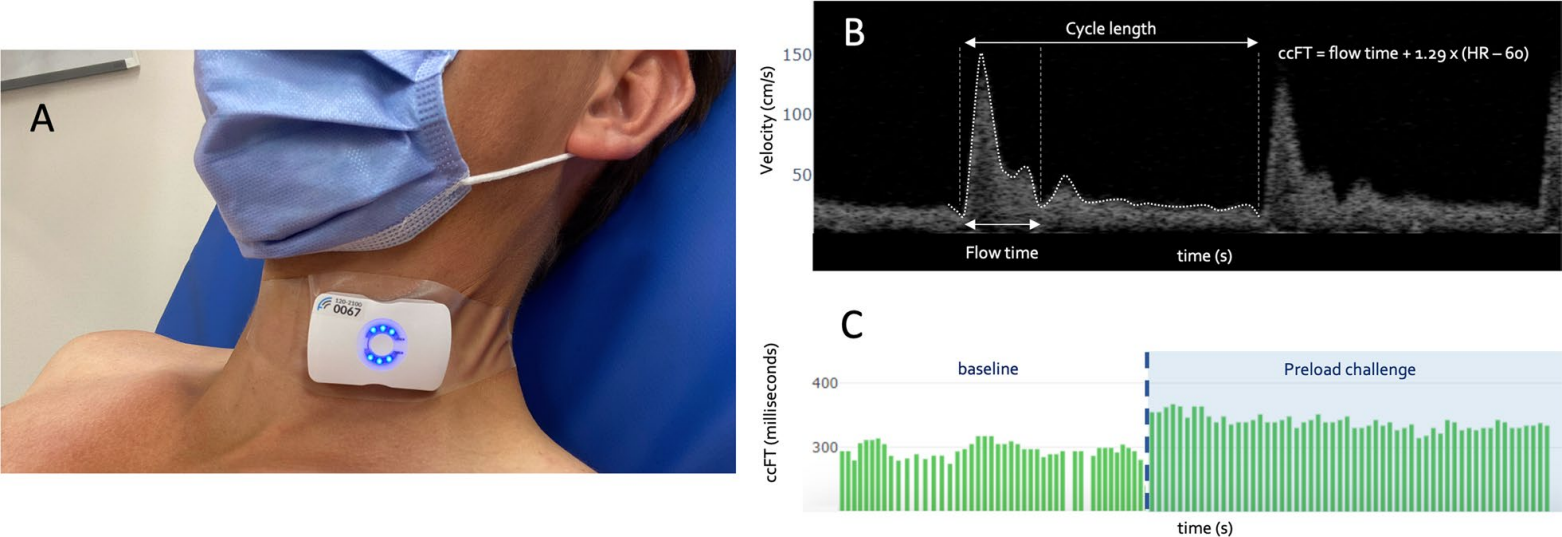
The wearable Doppler ultrasound and its interface. A The device on a healthy volunteer. B Carotid Doppler spectra showing calculation of carotid corrected flow time (ccFT) by the equation of Wodey. Velocity is on y-axis in centimeters per second (cm/s) and time on x-axis in seconds (s); heart rate (HR) is calculated from cycle length. C Example of a preload challenge shown on the graphical user interface of the wearable Doppler. Each green bar represents the ccFT of a single cardiac cycle; the *y*-axis is ccFT in milliseconds and the *x*-axis is time in seconds
